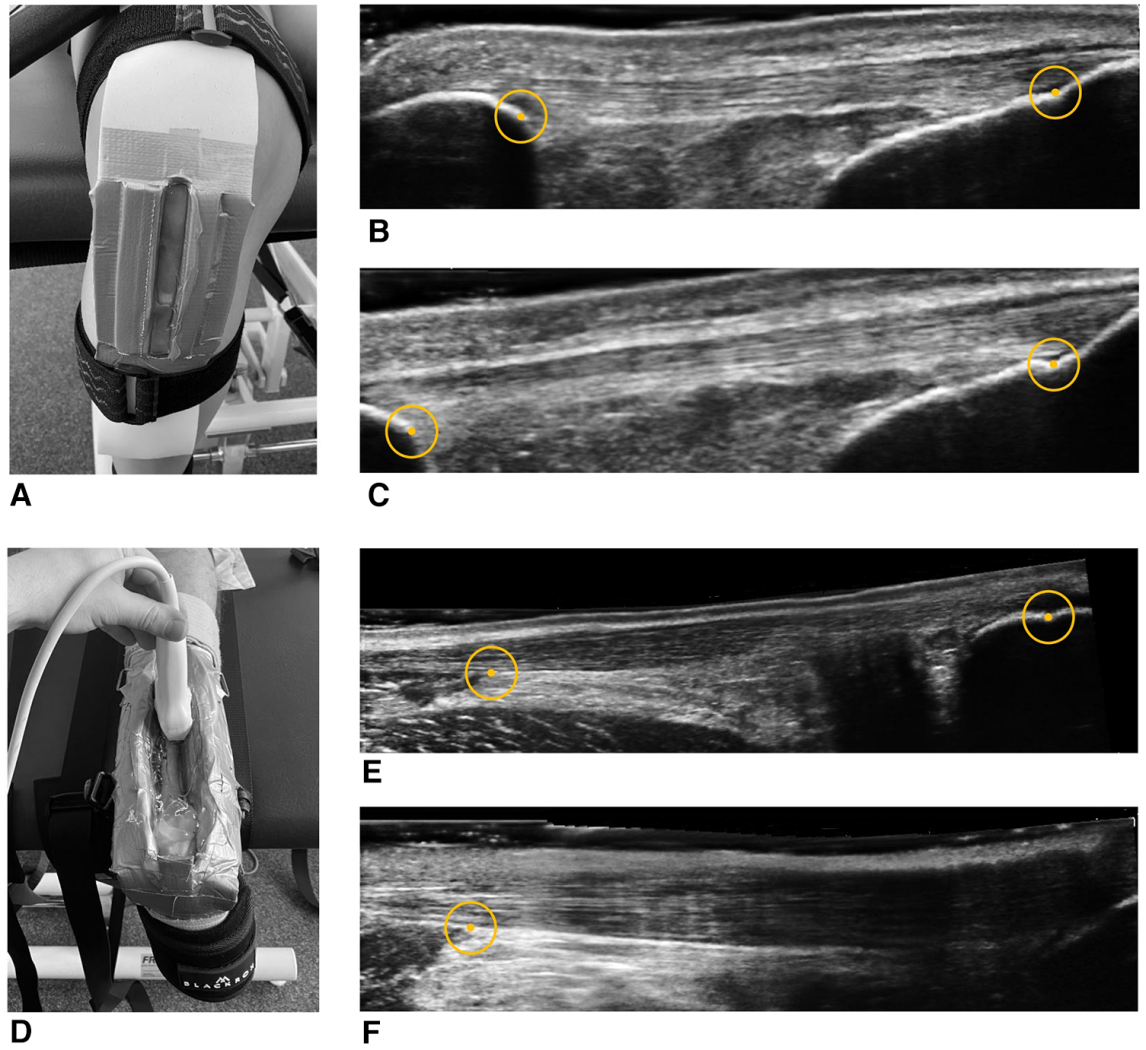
Self-customized fixable brace and anatomical reference points for ultrasound imaging and length measurement of the patellar and Achilles tendons. Ultrasound images of the patellar (A) and Achilles tendons (D) at rest (B,E) and maximal load (C,F), respectively. For point-to-point length measurements, the circles show the anatomical reference points (apex patella, tuberosity tibiae, M. soleus, and calcaneus notch). (F) Example of the common unclear reference point of the calcaneus at the maximal load measurements.
